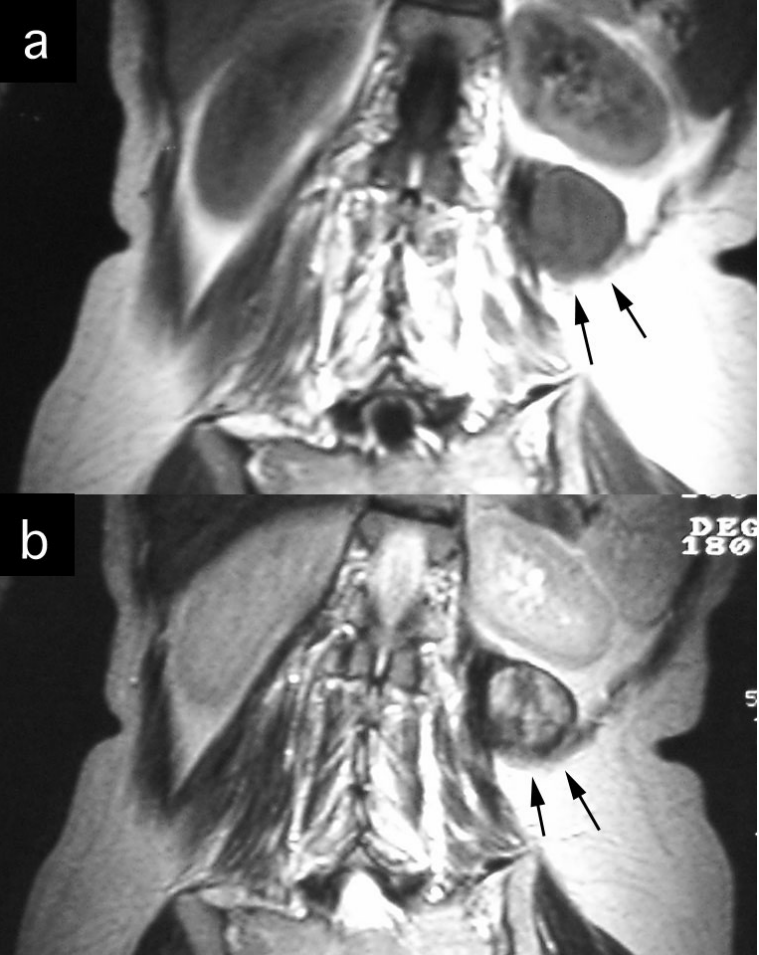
Coronal magnetic resonance imaging demonstrating the tumor beneath the left kidney.
